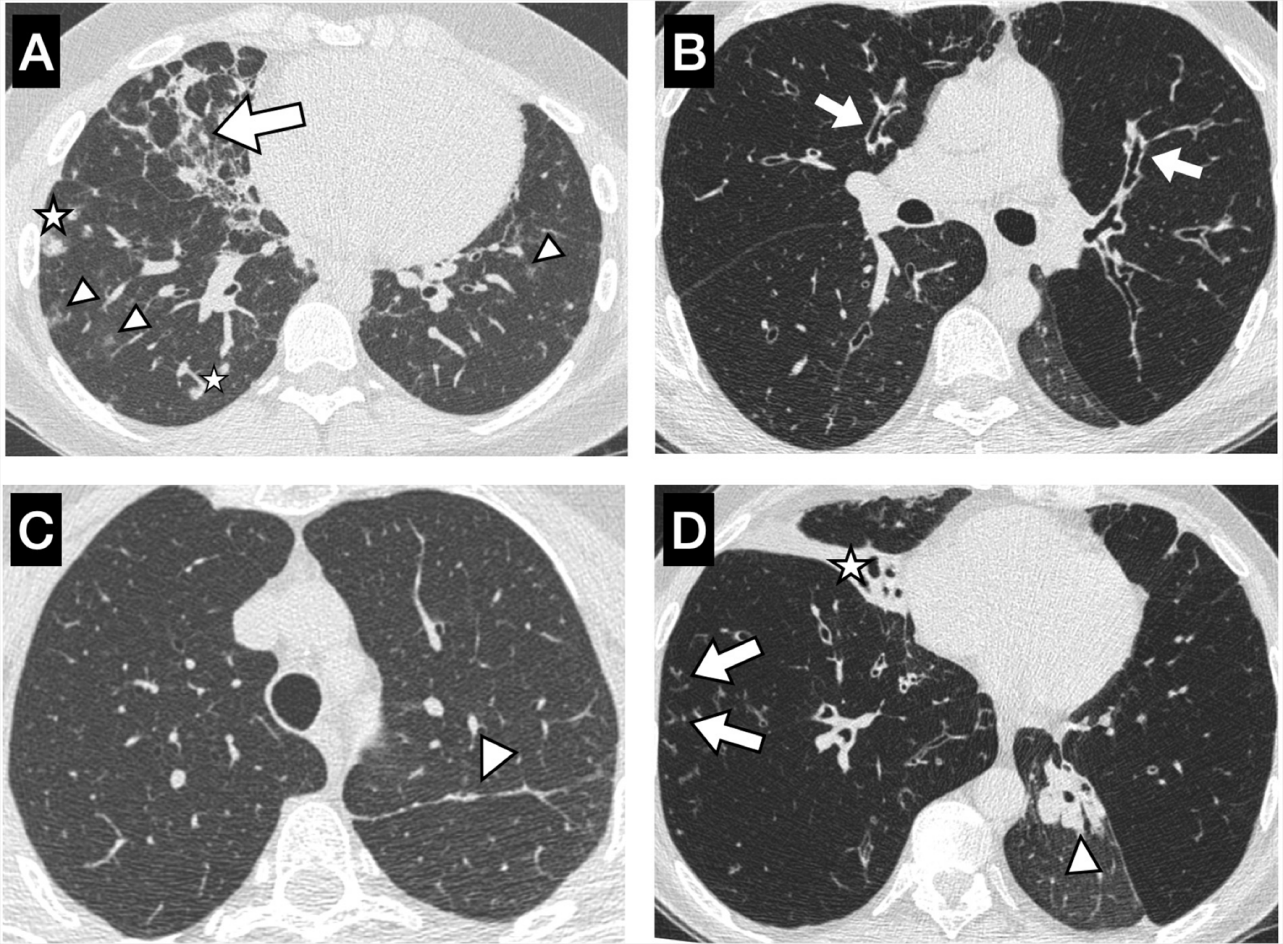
Chest CT from 4 different patients of our study with some of the evaluated parameters. (A) GLILD patient with multiple bilateral ground-glass nodules <5 mm (arrowheads), solid nodules (stars), and mixed (inflammatory and fibrotic) lines (arrow). (B) Non-GLILD patient with diffuse mild bronchial wall thickening and mild bronchiectasis (arrows) with air trapping predominantly in the upper lobes and partial atelectasis of left lower lobe. (C) Non-GLILD patient with linear parenchymal band in the left upper lobe (arrowhead). (D) Non-GLILD patient with mucus plugging in large airways of the left lower lobe with >50% atelectasis of the same lobe (arrows), complete atelectasis of the middle lobe (star), tree in bud pattern in the right lower lobe (arrows), and air trapping on the left upper and right lower lobes. GLILD, granulomatous–lymphocytic interstitial lung disease.
